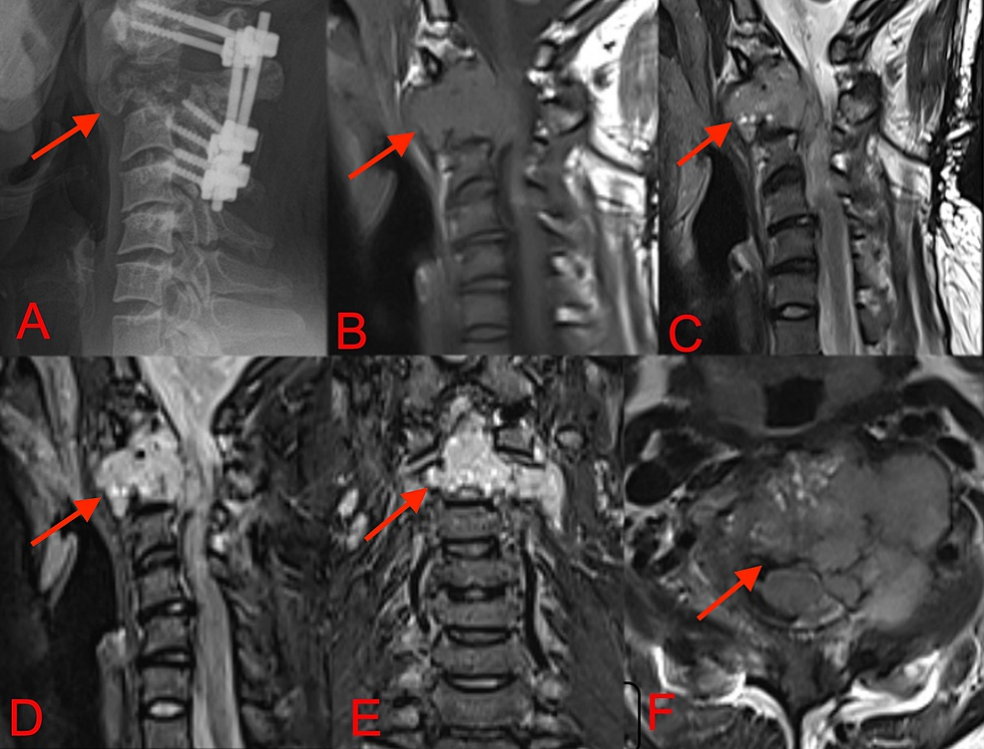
Three months after discontinuation of Denosumab, lateral radiograph (A) and sagittal T1 (B), T2 (C) and STIR (D, E, F) MRI showed a lytic, destructive lesion with similar signal characteristics as previous scan suggesting recurrence of the expansile tumor with destruction of C2 vertebral body and resultant compression of spinal cord with paraspinal soft tissue extension. STIR: short tau inversion recovery, MRI: Magnetic Resonance Imaging
